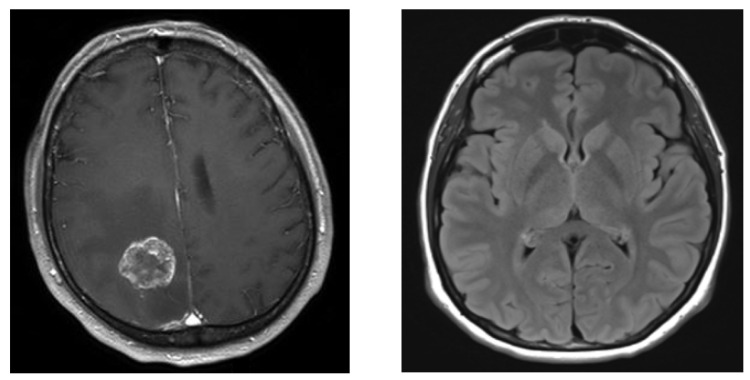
MR images of two classes: malignant (left) and benign (right) tumors.
